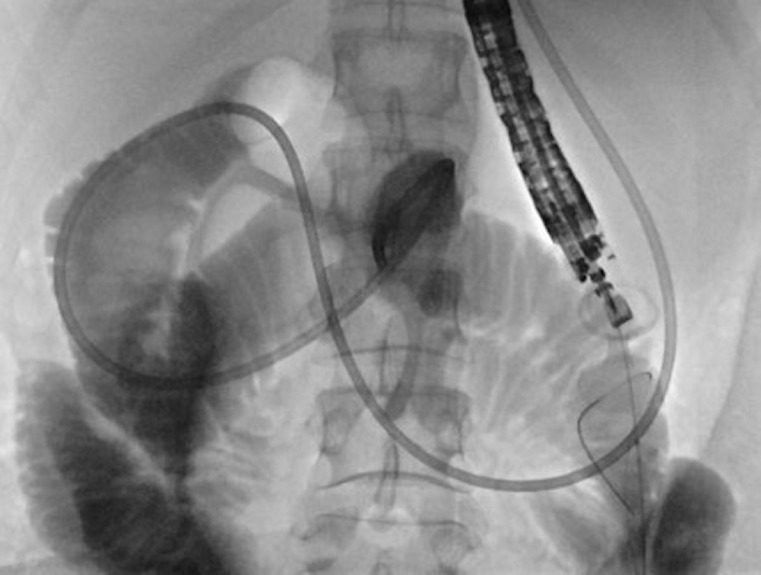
Fluoroscopy image of endoscopic ultrasound-guided creation of a gastroenterostomy with a 15 mm × 10 mm lumen-apposing metal stent.
